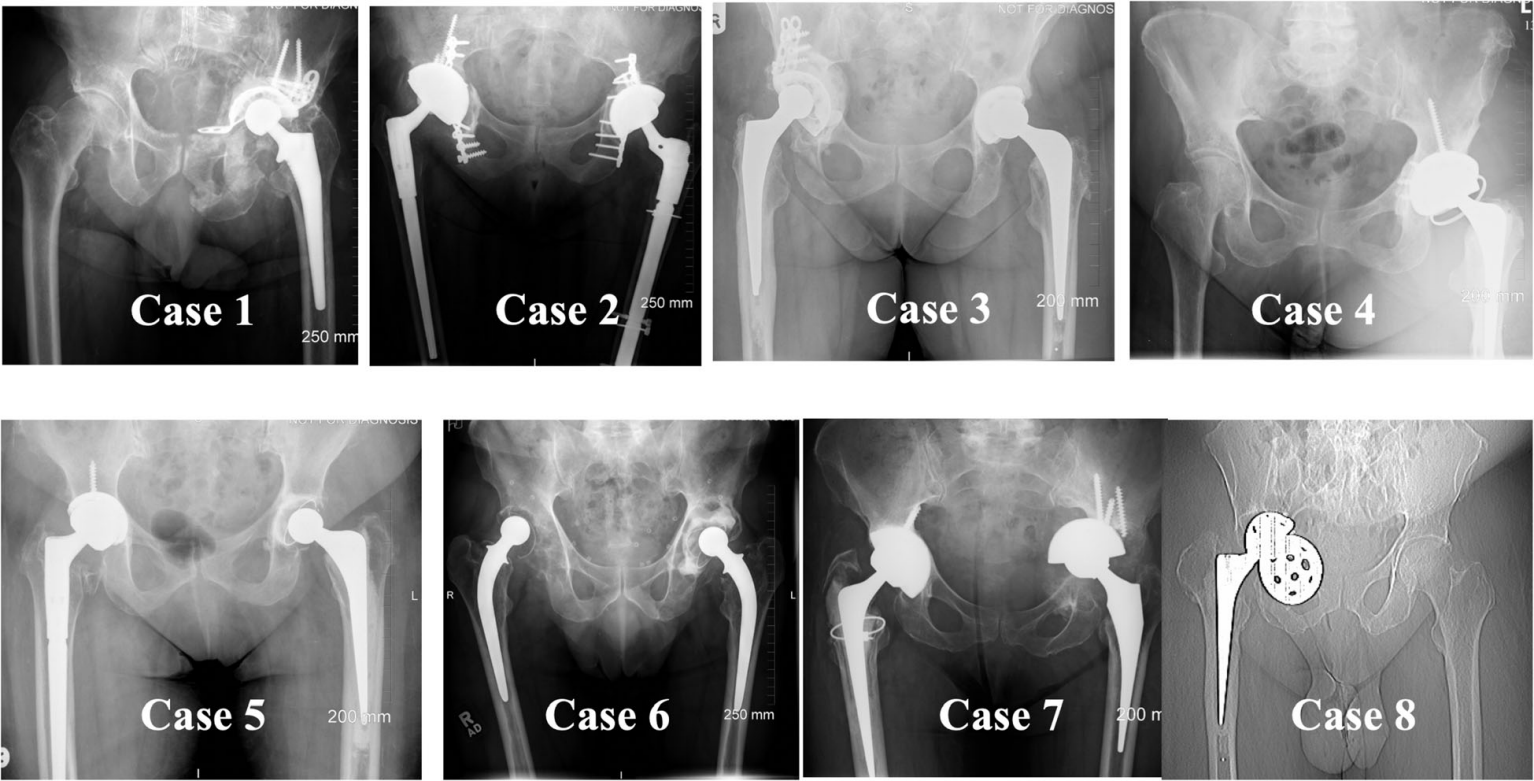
Pre-operative radiographs of the 8 patients included in the study. The acetabular defects were classified as Paprosky IIIB and beyond, there were three pelvic discontinuities (cases 2, 7 and 8)
